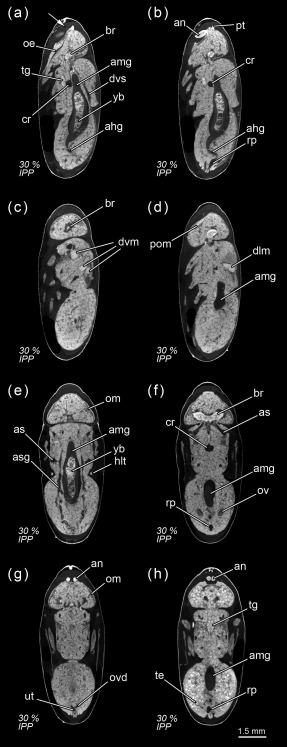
Calliphora vicina*, micro‐CT‐based virtual sections of puparia 72 hr after pupariation (30% of the total intra‐puparial period, IPP), reared at 24°C. (a) medial sagittal section. (b) latero‐medial sagittal section. (c) lateral sagittal section. (d) lateral sagittal section. (e) dorsal horizontal section. (f) female, ventral horizontal section. (g) female, ventral horizontal section. (h) male, ventral horizontal section. ahg, adult hindgut; amg, adult midgut; an, antennae; as, air sac; asg, adult salivary gland; br, brain; cr, crop; dlm, dorsal longitudinal muscles; dvm, dorsoventral muscles; dvs, dorsal vessel; hlt, halters; oe, oesophagus; om, ommatidia; ov, ovaries; ovd, oviduct; pom, ptilino‐oesophagal muscle; pt, ptilinum; rp, rectal pouch; te, testes; tg, thoracic ganglion; ut, uterus; yb, yellow body
